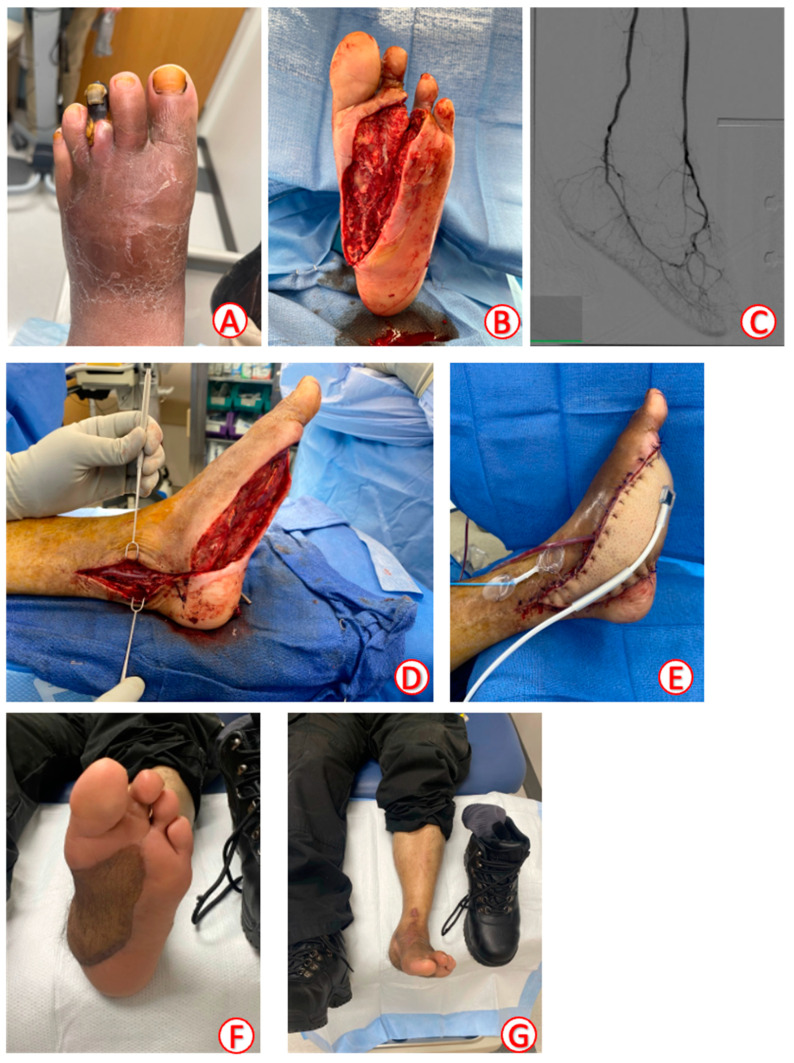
A 53-year-old male with a past medical history of type II diabetes mellitus with peripheral neuropathy A1c 9.3% who presents to the clinic with a left foot infection, abscess, and gas gangrene. (A) Patient presents to the clinic with infection of the L foot and gangrene of the 3rd toe. Patient is admitted to the limb surgical service. Patient receives a CT scan, which confirms gas gangrene and shows changes corresponding to osteomyelitis. (B) Photograph post incision and drainage of left foot and open partial third ray amputation. Patient presents with a large plantar foot defect measuring approximately 13 × 7 cm in size, extending from second webspace anteriorly and continuing plantarly and medially to the medial aspect of calcaneal region with exposed intrinsic muscles of the foot. Post-debridement cultures are positive for polymicrobial infection. Biopsy of the left toe is taken and reported to show evidence of acute osteomyelitis. Patient receives four additional debridements prior to free flap. (C) Photograph of patient’s diagnostic angiogram showing 2-vessel run-off with widely patent anterior tibial (AT) artery, which continue onto the foot as a dorsalis pedis artery (arrow), widely patent posterior tibial (PT) artery which continues onto the foot as plantar vessels, and intact pedal arch (arrow). Return to the OR for second debridement; photograph of post-excisional debridement (obtained from chart). (D) Intraoperative photograph of recipient site dissection. Incision placed 2 cm posterior to medial malleolus where strong doppler signal is heard from the posterior tibial artery. Posterior tibial artery measures 2.5 mm in caliber with evidence of moderate calcifications throughout the vessel. (E) Intraoperative photograph of free anterolateral (ALT) flap with end-to-side anastomosis to PT. Implantable Cook Doppler and Vioptix placed for postoperative monitoring. (F) Follow-up in clinic 9.6 months post-op showing well-healed free flap. (G) Follow-up in clinic 9.6 months post-op showing patient is ambulating with regular footwear.
